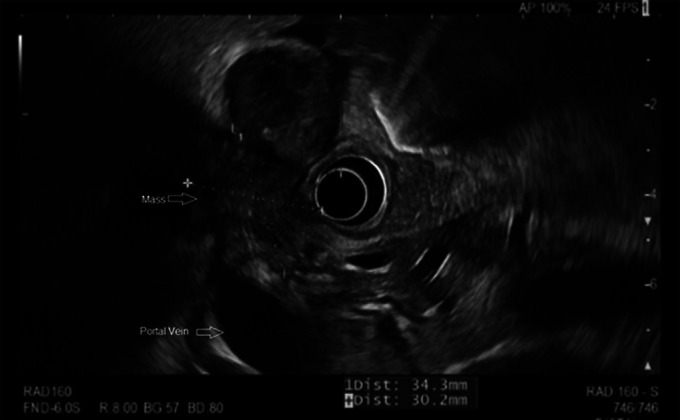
Endoscopic ultrasound image of the common hepatic/gallbladder mass.
